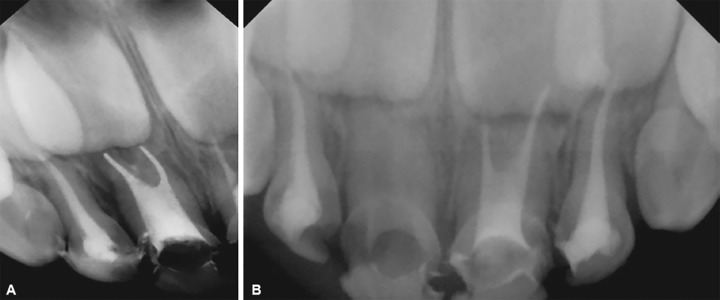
(A) Postobturation radiograph with 51; and (B) postobturation radiograph with 61, 62
